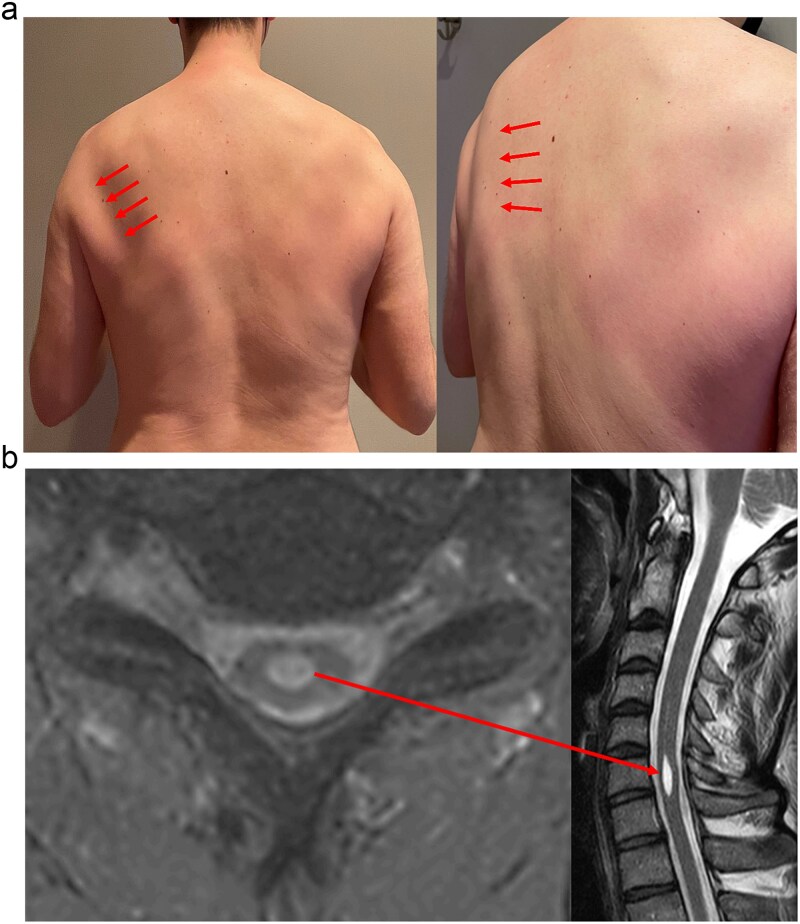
(a) Clinical image of scapula alata with slight winging of the left scapula (marked by arrows). (b) MRI of the cervical spine showing cervical syringomyelia at the level of the sixth cervical vertebra with a craniocaudal extension of ~20 mm. The syringomyelia appears hyperintense on the axial mFFE image (left) and sagittal T2-weighted image (right); the lesion is marked with an arrow.
